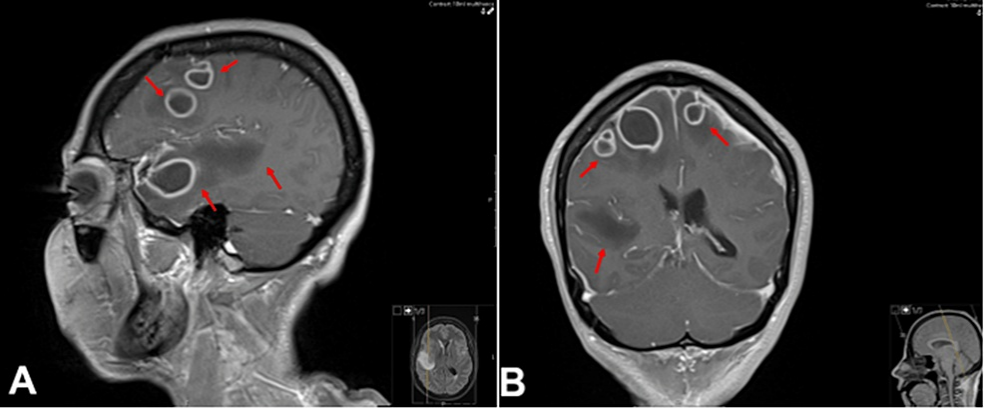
MRI shows multiple right intra-axial lesions with surrounding edema (red arrows) in bilateral frontal lobes and right anterior temporal lobe. (A) Sagittal plane; (B) coronal plane MRI: magnetic resonance imaging.
